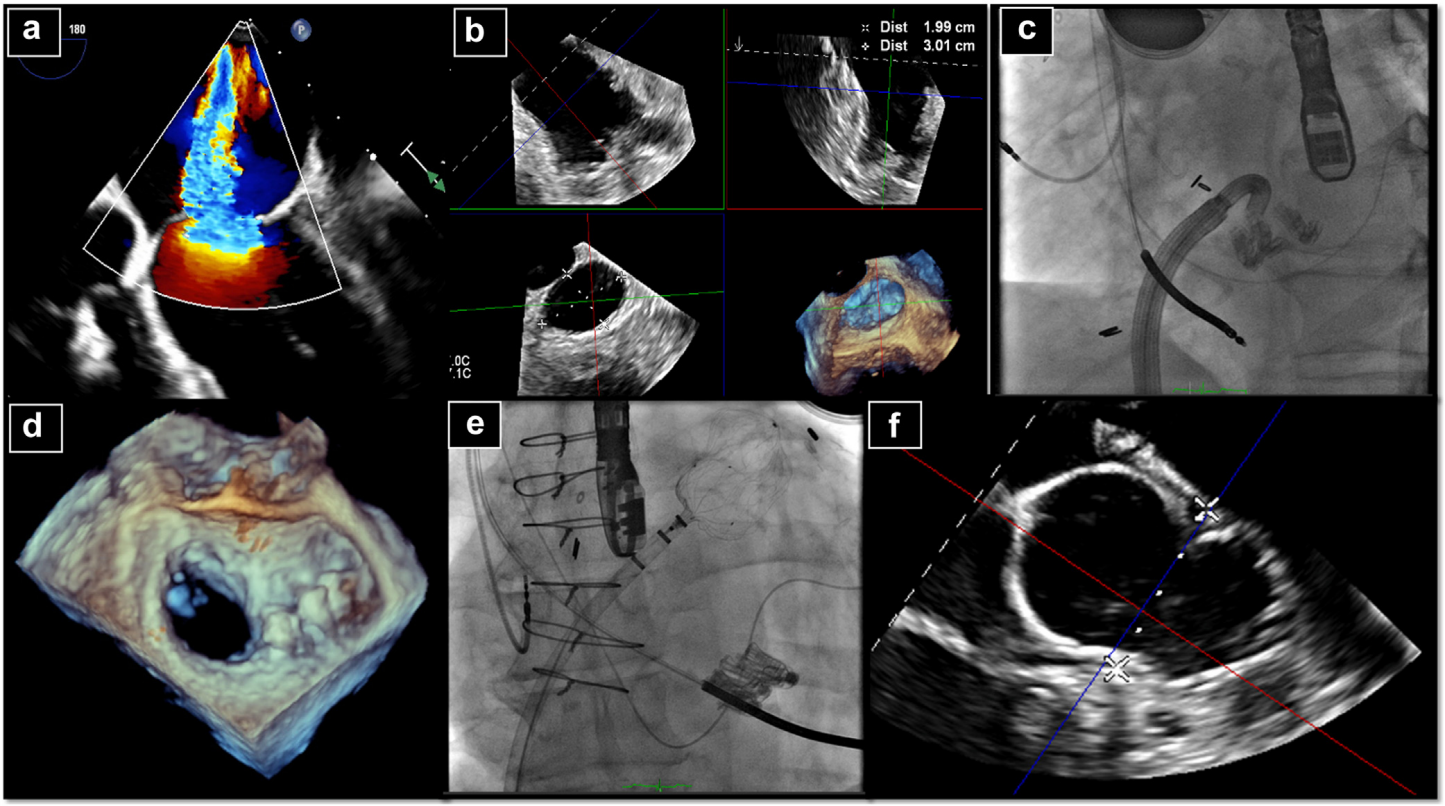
Example of combined M-TEER ​+ ​LAAO. (a and b) Use of TEE prior to M-TEER to assess severity and mechanism of MR and size for Watchman placement; (c and d) placement of three Edwards Pascal/ACE devices; (e and f) successful placement of Watchman FLX Pro device via the same TSP and femoral venous access site used for M-TEER, using fluoroscopy and TEE for guidance Abbreviations: LAAO, left atrial appendage occlusion; M-TEER, mitral transcatheter edge-to-edge repair; MR, mitral regurgitation; TEE, transesophageal echocardiography; TSP, transseptal puncture.
